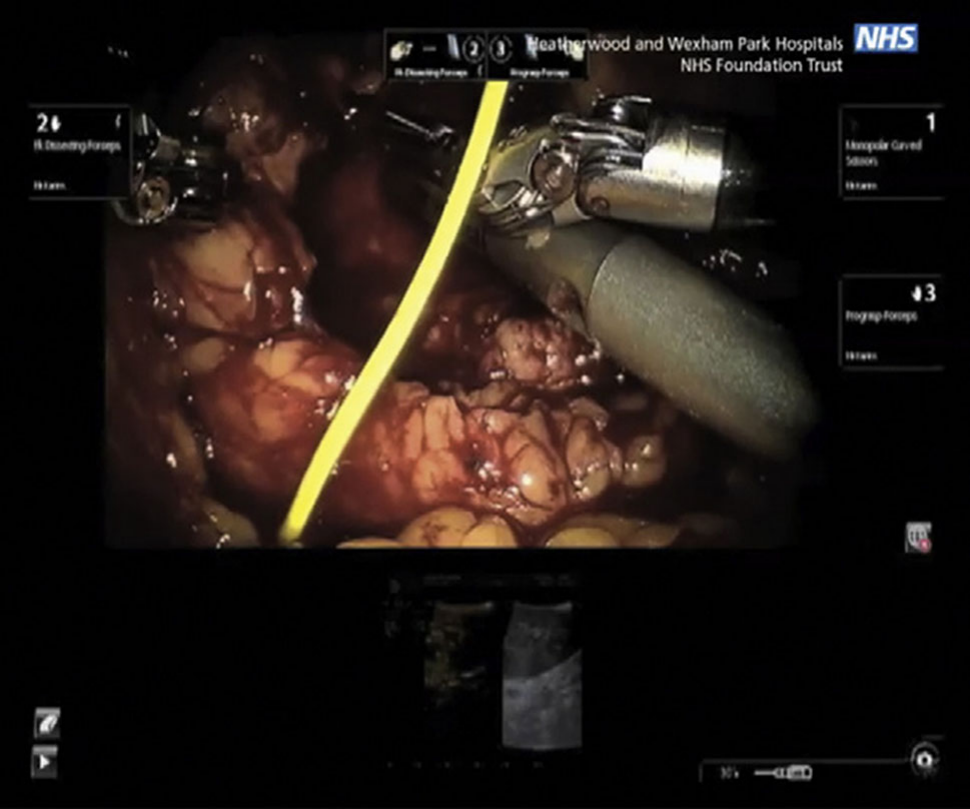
Using the robotic ProGrasp forceps the surgeon manipulates the robotic ultrasound probe over the kidney, to visualize the position of the tumor (B-mode ultrasound), and assess blood flow (using CEUS). The operating surgeon views the ultrasound images in the TilePro mode on the robot console. The B-mode ultrasound image is on the right, while the CEUS image is on the left of the TilePro image [5]
